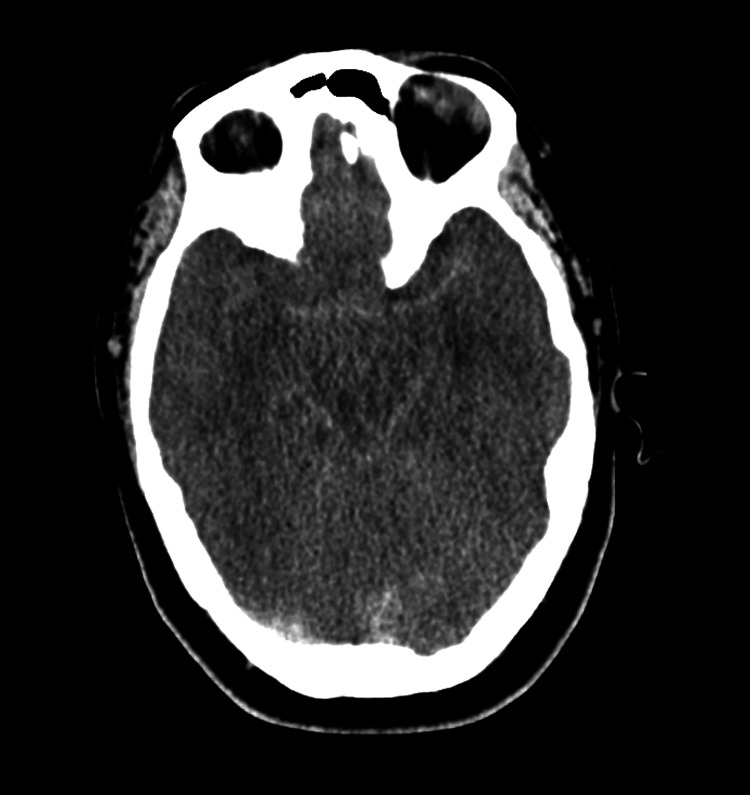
CT head orbital slice Diffuse cerebral edema with loss of gray–white differentiation and sulcal effacement is observed, along with effacement of the basal cisterns, consistent with increased intracranial pressure.
